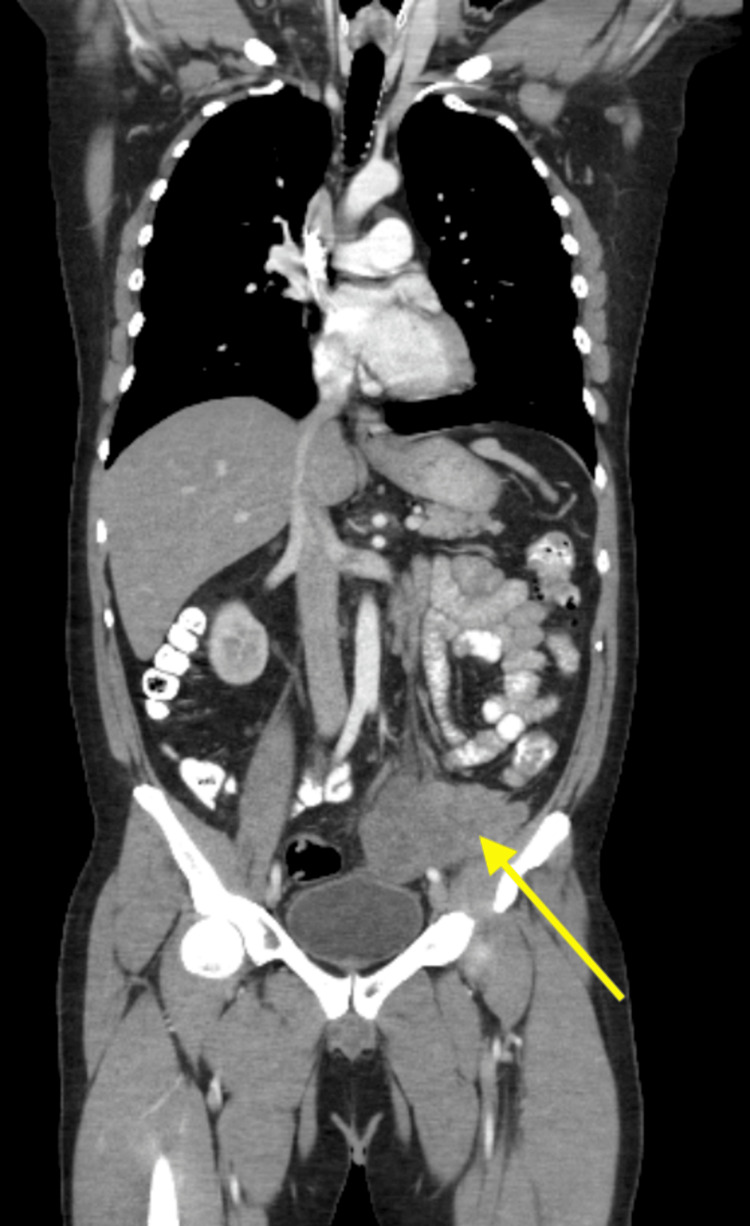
CT scan of the chest, abdomen, and pelvis showing interval decrease in the size of left pelvic mass (yellow arrow)
